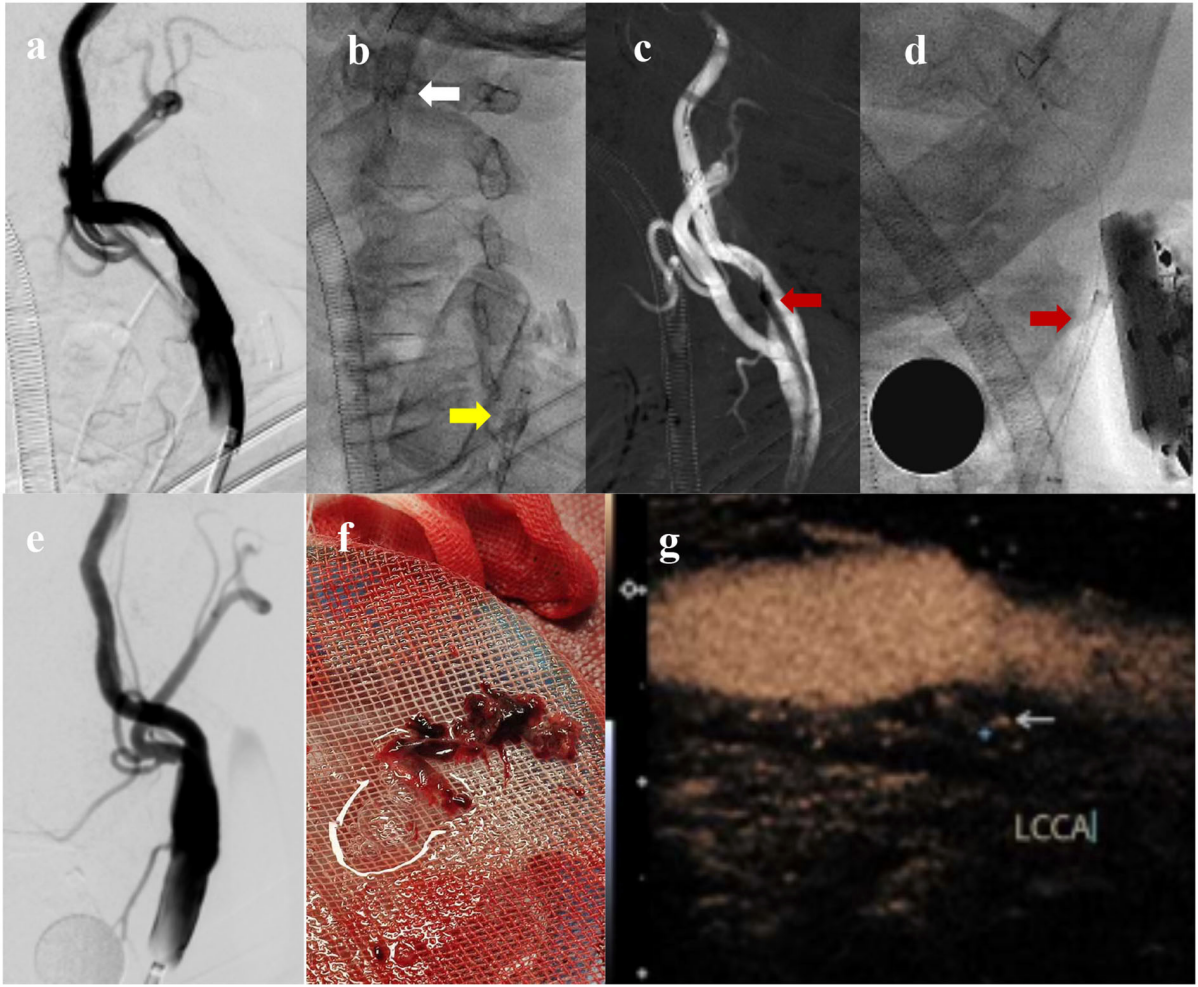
Carotid DSA before aspiration (a); The FlowGate^2^ balloon guide catheter (yellow arrow) is placed in the left common carotid artery and the Emboshield NAV6 embolic protection system (white arrow) is placed in the distal segment of the internal carotid artery (b); The Mach1 guide catheter (red arrow) is inserted to aspirate CFFT (c); Ultrasound probe depressing Mach1 tip (red arrow) to face the thrombus (d); Carotid DSA after aspiration (e); Massive thrombus has been aspirated (f); Postoperative contrast-enhanced ultrasound showed unstable plaque with no evidence of CFFT (g).
